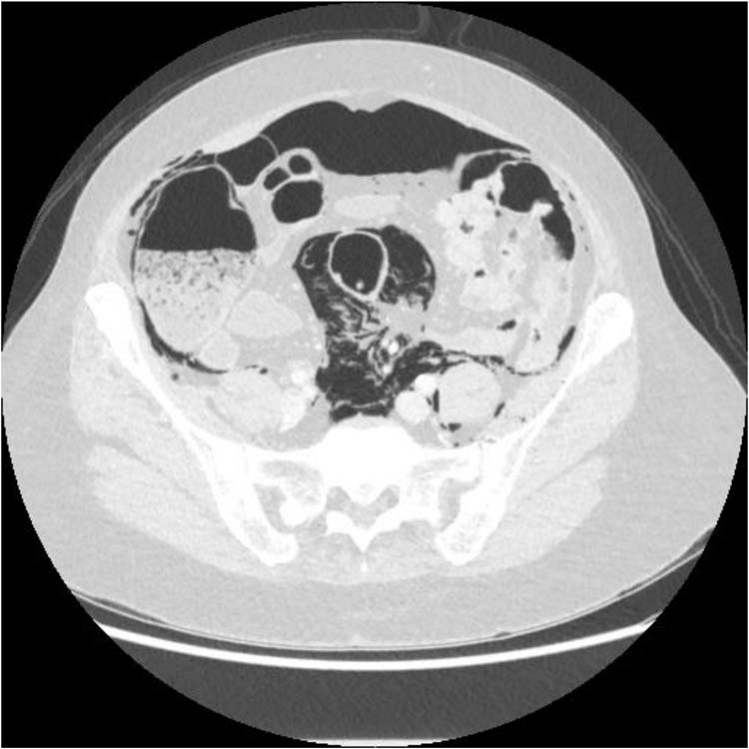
Axial CT showing extensive intra- and extraperitoneal free gas.
